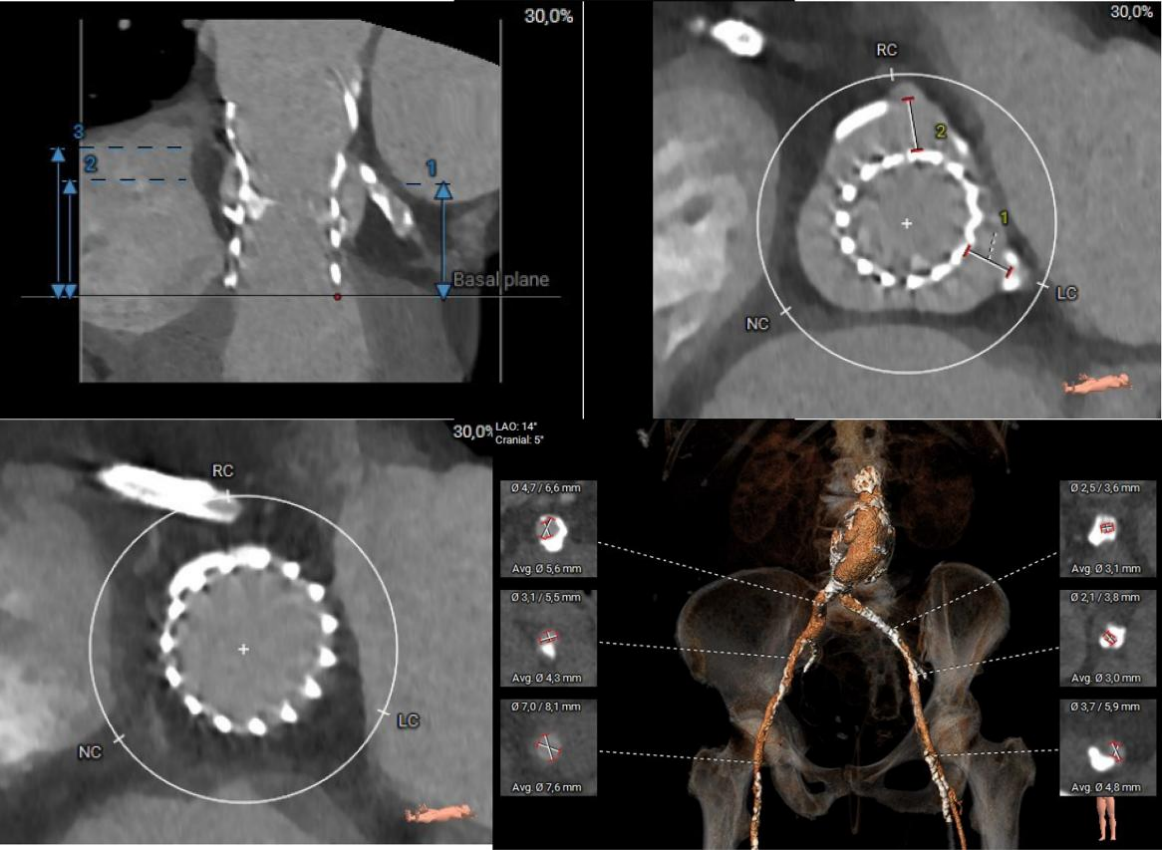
Pre-procedural CT scan with the index Evolut R 26 mm THV in relation to the aortic root and coronary ostia as well as access vessels. Upper left: depicts a stretched image of aortic root and index THV valve with measurement from the bottom of the THV stent frame to (1) LCA ostium (26.3 mm), (2) RCA ostium (27.6 mm), and (3) ST-junction (35.1 mm). Upper right: cross-section at the level of the offspring of the coronary arteries. VTC was measured to (1) 7.9 mm for the LCA and (2) 8.7 mm for the RCA, respectively. Bottom left: cross-section at the level of the STJ. Note that there is no space in between aorta and the expanded index valve. Bottom right: depicts the femoral and iliac arterial vessels. The minimal average diameter in the right vessel was 4.3 mm at the level of the internal iliac artery and in the left vessel 3.0 mm at the level of the common iliac artery. Note the aortic abdominal aneurysm.
